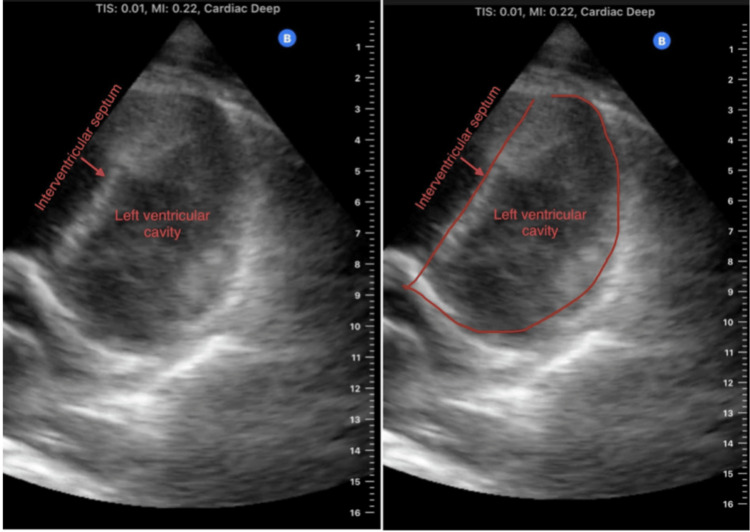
Cardiac POCUS using the short-axis left parasternal view showing flattening of the interventricular septum and giving rise to the D-shaped left ventricle (D-sign), which is seen in patients with right ventricular strain. POCUS, point-of-care ultrasound
